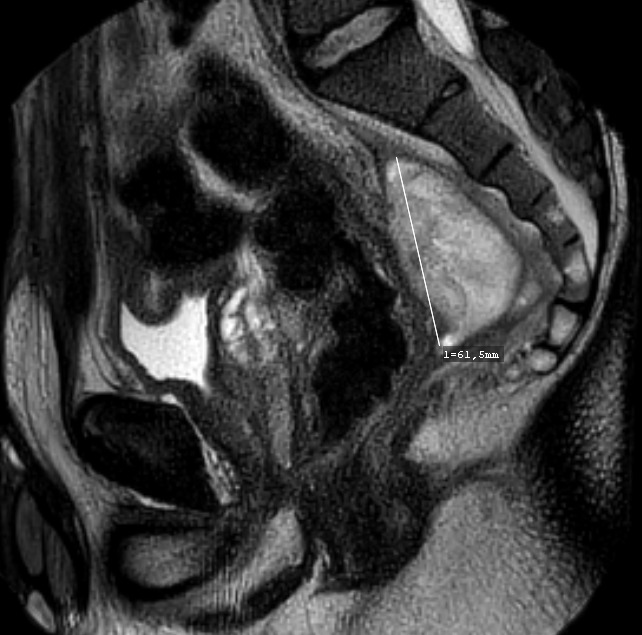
Sagittal T2-weighted pelvic magnetic resonance imaging shows perianal abscess
